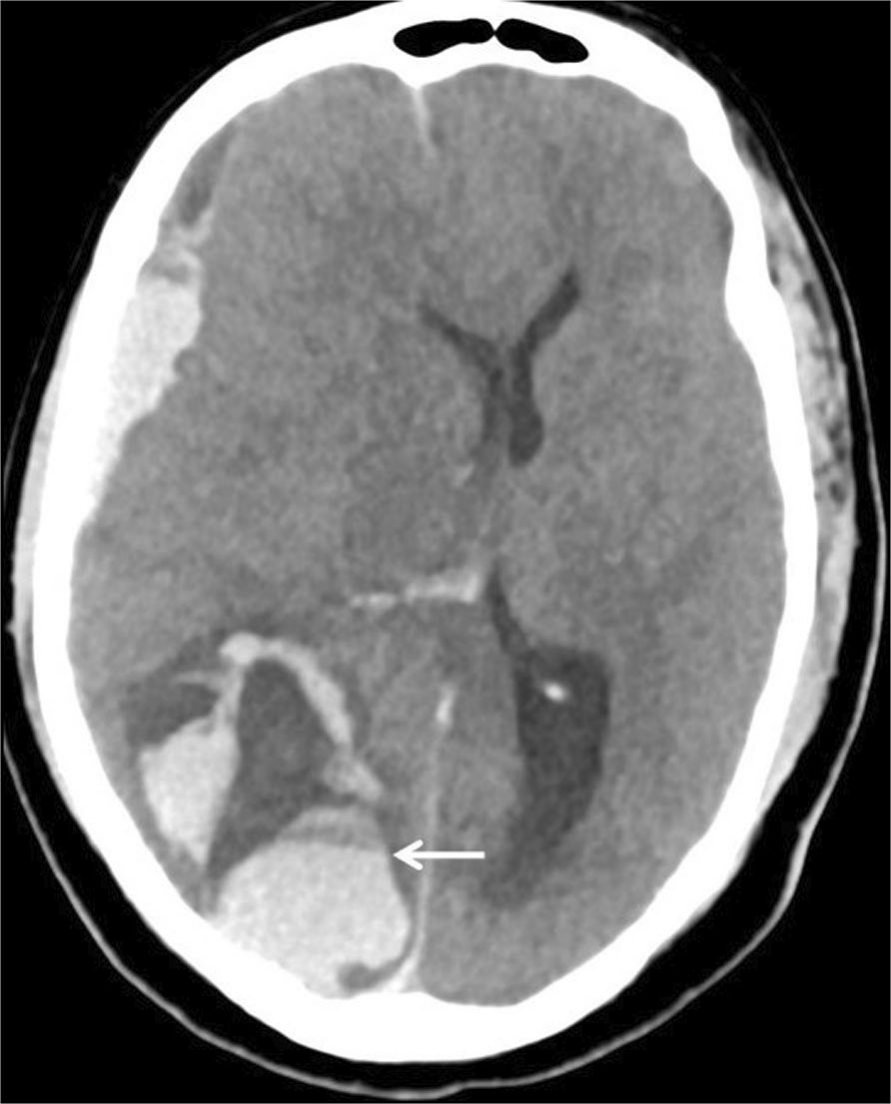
Parenchymal hemorrhage. 43-year-old man with COVID-19 transferred to ICU, developed acute myocardial infarction, and received thrombolytic therapy. He suddenly deteriorated and was found with bilaterally fixed and dilated pupils. Axial non-contrast CT of the brain revealed acute subdural hemorrhage, large occipito-parietal intraparenchymal hematoma with blood-fluid level (arrow)
